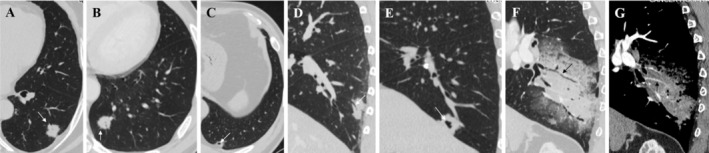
Morphological/internal features of pulmonary invasive mucinous adenocarcinoma on CT images. (A,E) Lobulated sign. (A,B) Spiculated sign. (C) Vacuole sign, with the diameter of air‐containing space ≤ 5 mm. (D) Air bronchogram sign, referring to the smooth bronchus passing through the consolidation area. (E) Cystic cavity, with the diameter of air‐containing space >5 mm. (A,C,D,E) Pleural attachment. (F) Dead branch sign, referring to the dilatation, stiffness, or narrowing of bronchi passing through the consolidation area. (G) Angiogram sign, named after the obviously enhanced branching pulmonary vessels passing through the low‐density consolidation area on the enhanced CT.
